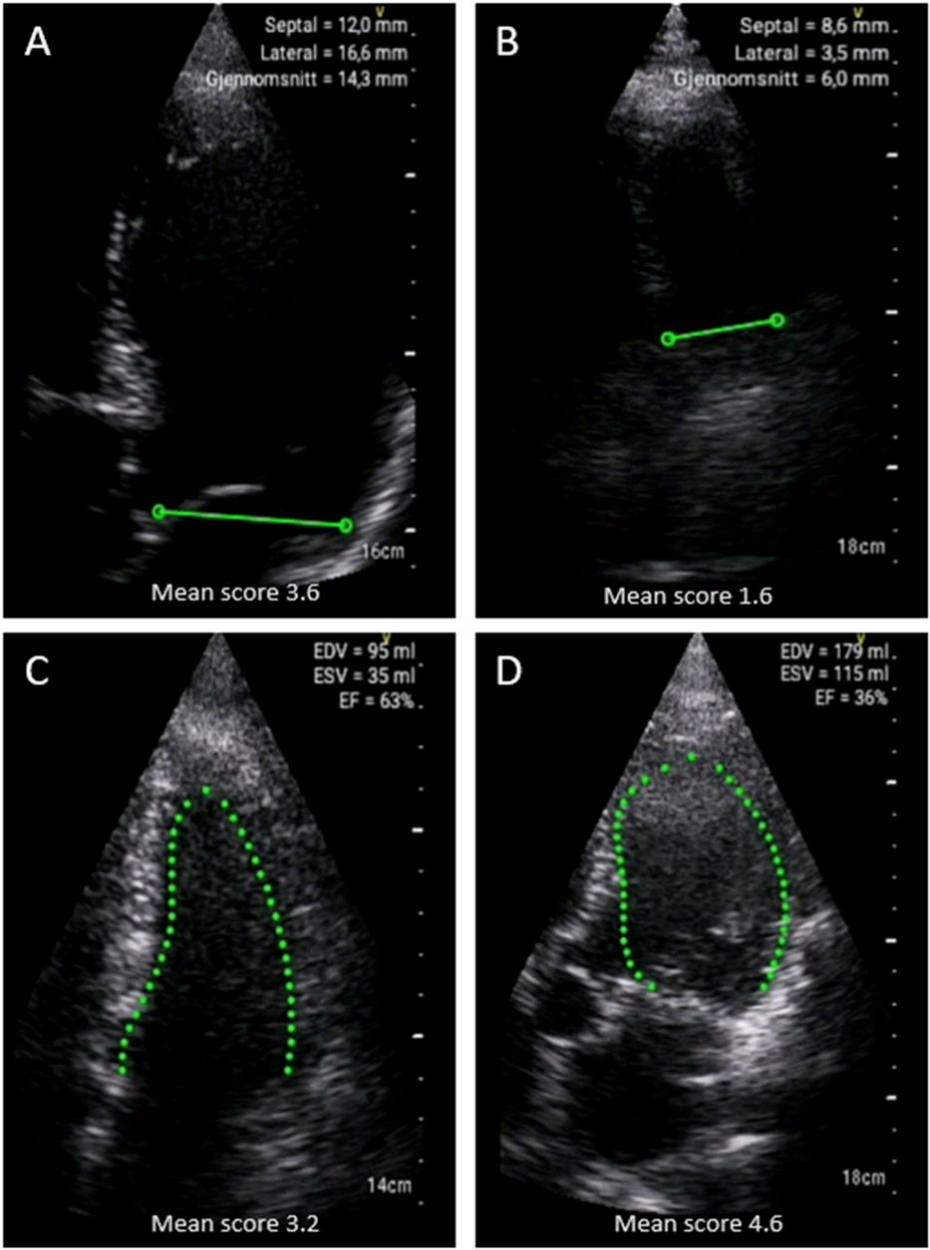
Examples of ultrasound recordings in patients with correct and incorrect diagnoses by GPs. (*A*) Correct non-HF diagnosis with normal autoMAPSE. (*B*) Incorrect HF diagnosis with reduced autoMAPSE. (*C*) Correct non-HF diagnosis with normal autoEF. (*D*) Incorrect HF diagnosis with reduced autoEF.EDV, end-diastolic volume; ESV, end-systolic volume. Explanation: ‘Gjennomsnitt’ in the upper panel is Norwegian for ‘average’.
